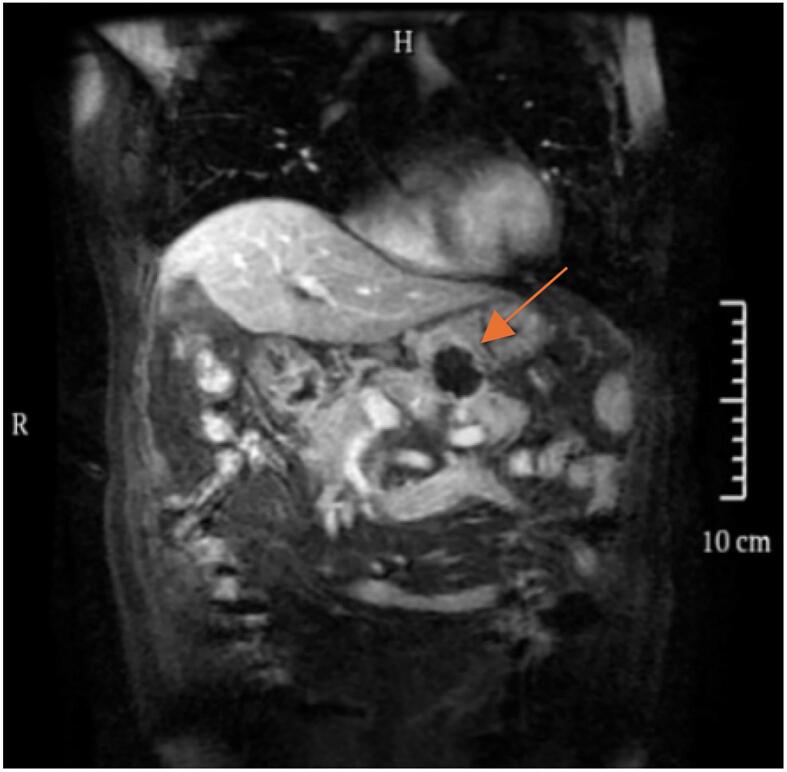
T2-weighted magnetic resonance imaging showing a space-occupying lesion measuring approximately 46 mm × 34 mm in the posterior peritoneum between the pancreas and the lesser curvature of the stomach.
